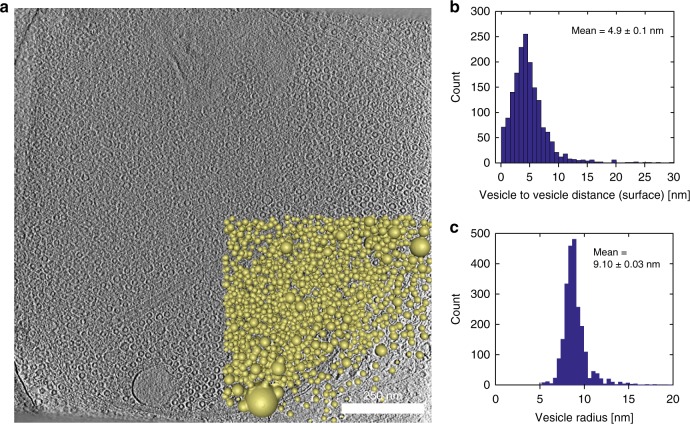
Cryo-electron tomogram of the tripartite droplets. a A mixture of 1 μM of SLP65_1-330_ and 1 μM of CIN85_Δ57_ together with 1 mM of SUVs was placed on a carbon grid and plunged frozen after incubation. The droplet shows a clear increase in concentration of vesicles toward its center with a steep fall-off at the edges. Golden spheres represent vesicles that were manually segmented for 3D-visualization in AMIRA 6.2 and processed using an in-house python script and Matlab. b Histogram of distances between vesicles with an average vesicle to vesicle distance of 4.92 ± 0.1 nm (mean ± SEM; *n* = 2349). c Histogram of the vesicle’s radii with a mean radius of 9.10 ± 0.03 nm (mean ± SEM; *n* = 1718). Source data are provided as a Source Data file.
